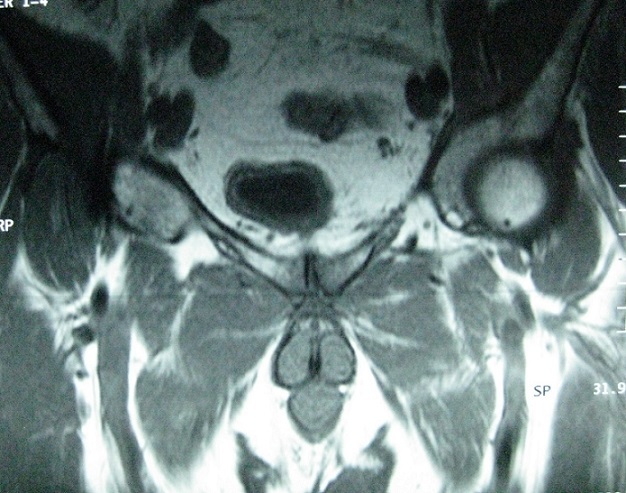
MRI of the pelvis: pubic symphysitis with inflammatory aspect of the major adductor muscles and thickening of the prepubic soft tissues
